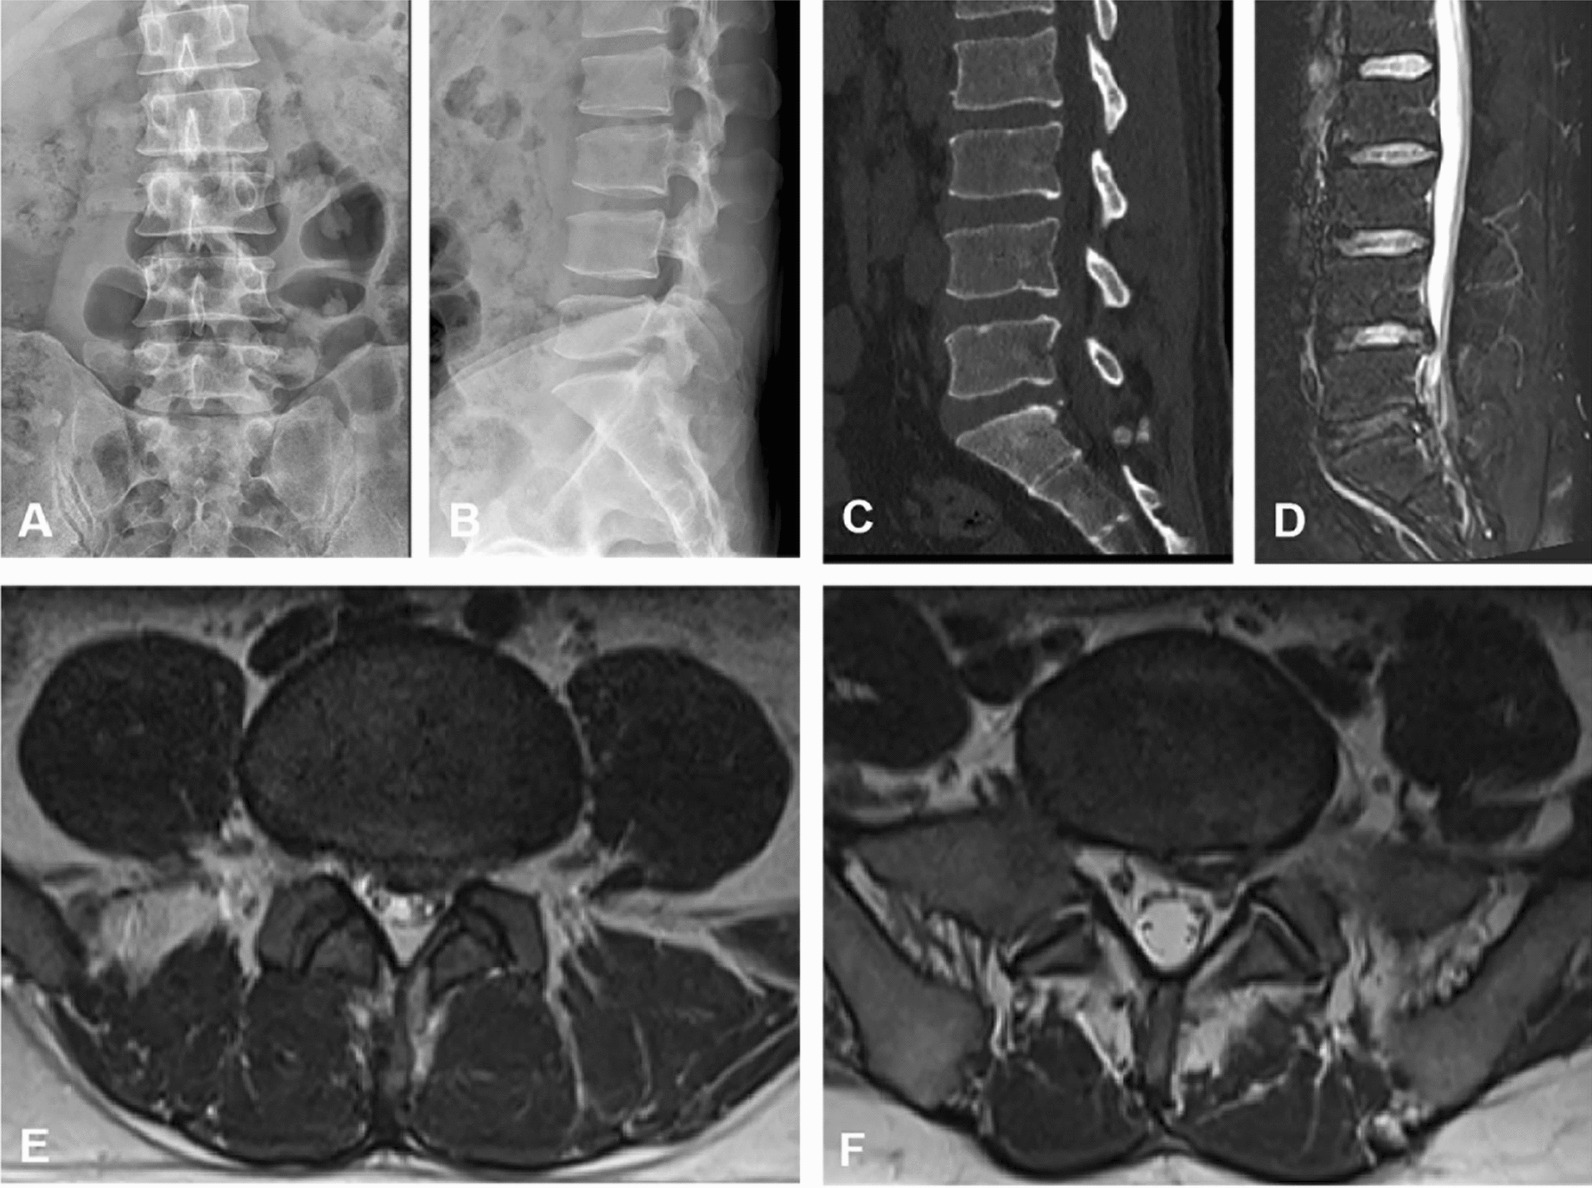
Preoperative radiological examination of a 48-year-old male patient with L4/5 and L5/S1 LDH. A–B: Frontal and lateral X-ray images. C: Sagittal CT image. D: Sagittal MRI image. E: Cross-sectional MRI image of L4–L5. F: Cross-sectional MRI image of L5-S1
